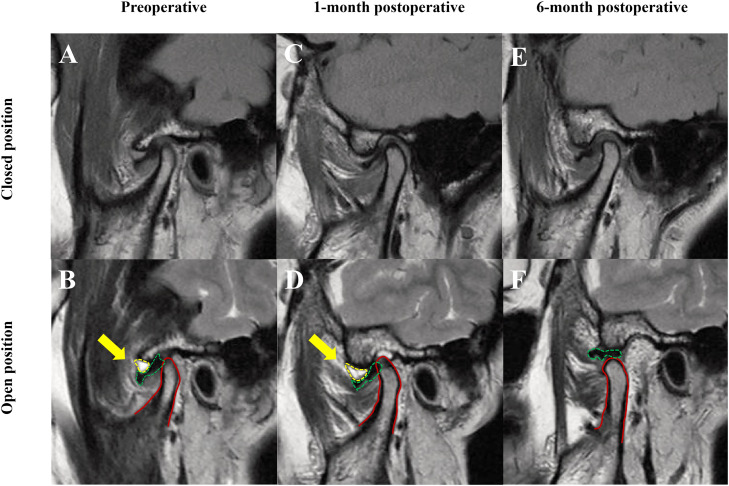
Changes in MRI before and after ARDE treatment. The patient exhibited notable fluid accumulation in the joint cavity prior to surgery, which increased 1 month after the administration of ARDE treatment, potentially due to the injection. However, after 6 months, no significant joint effusion was observed in the joint cavity of the patient (as indicated by the yellow arrow). The patient’s disc position transformed from anterior disc displacement without reduction to anterior disc displacement with reduction (as indicated by the green dashed line).
